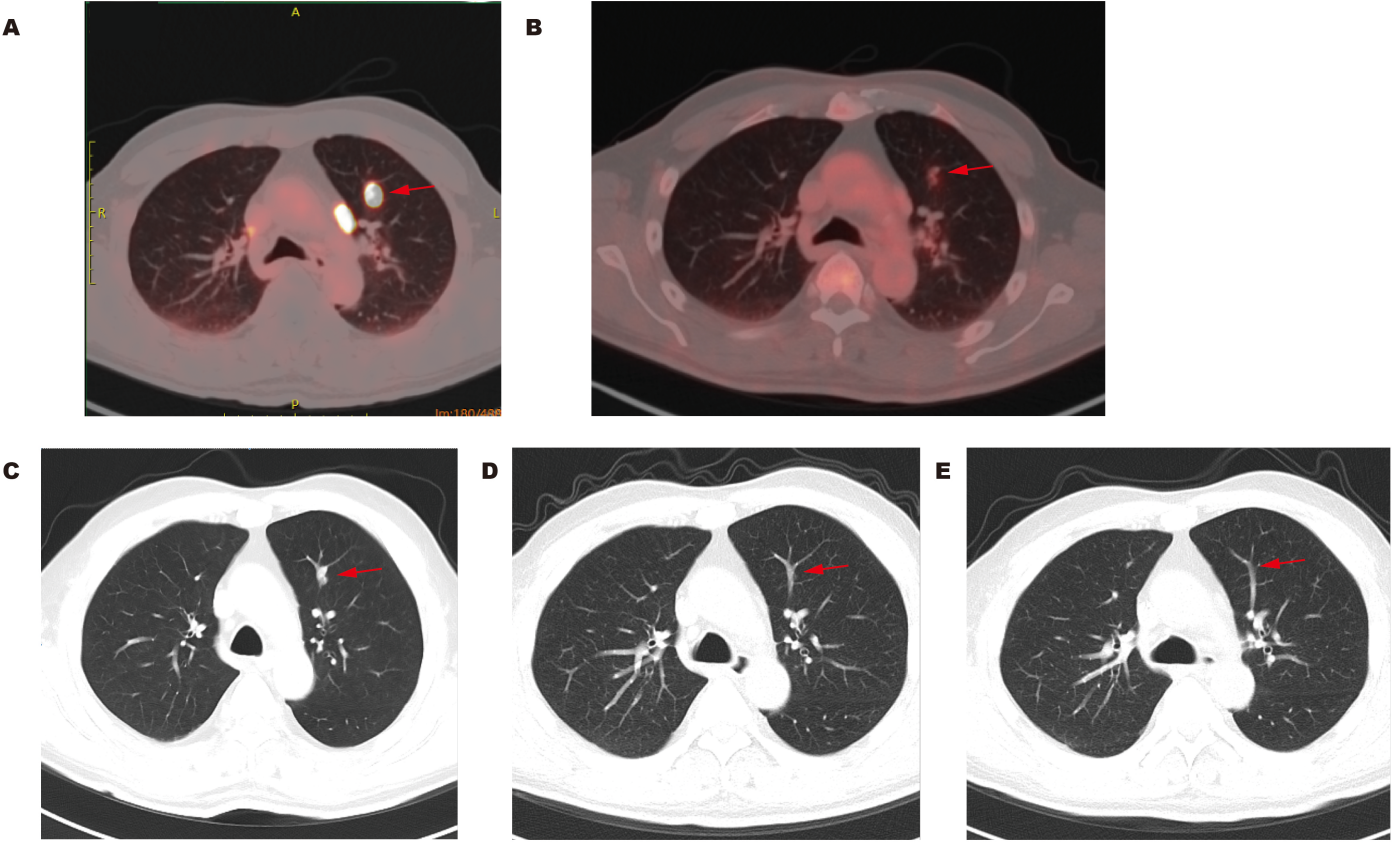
Representative PET/CT and CT images of lungs before and after treatment. (A) Lung metastasis (red arrow) showing markedly elevated PSMA uptake (SUVmax 21.2) before treatment; (B) Lung metastasis (red arrow) showing only mildly increased FDG uptake (SUVmax 2.2) before treatment; (C) CT image of the same lung metastatic site (red arrow) at the corresponding plane with PET/CT before treatment; (D) CT image showing a significant reduction in the same lung metastatic site (red arrow) at the corresponding plane after two cycles of treatment; (E) CT image showing a significant reduction in the same lung metastatic site (red arrow) at the corresponding plane after four cycles of treatment.
